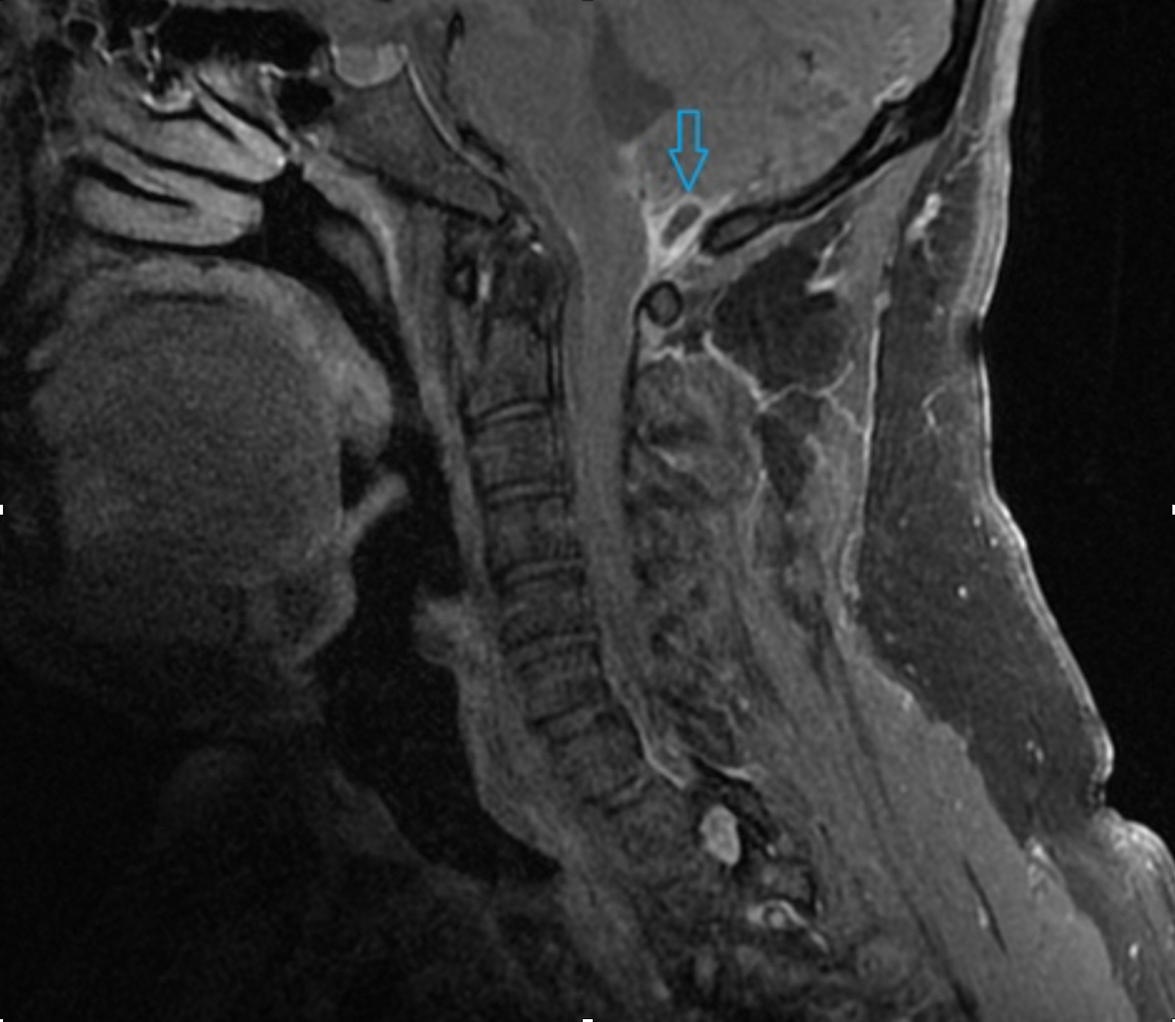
T1-weighted image with contrast showing leptomeningeal enhancement dorsal surface of the upper cervical cord (blue arrow).
